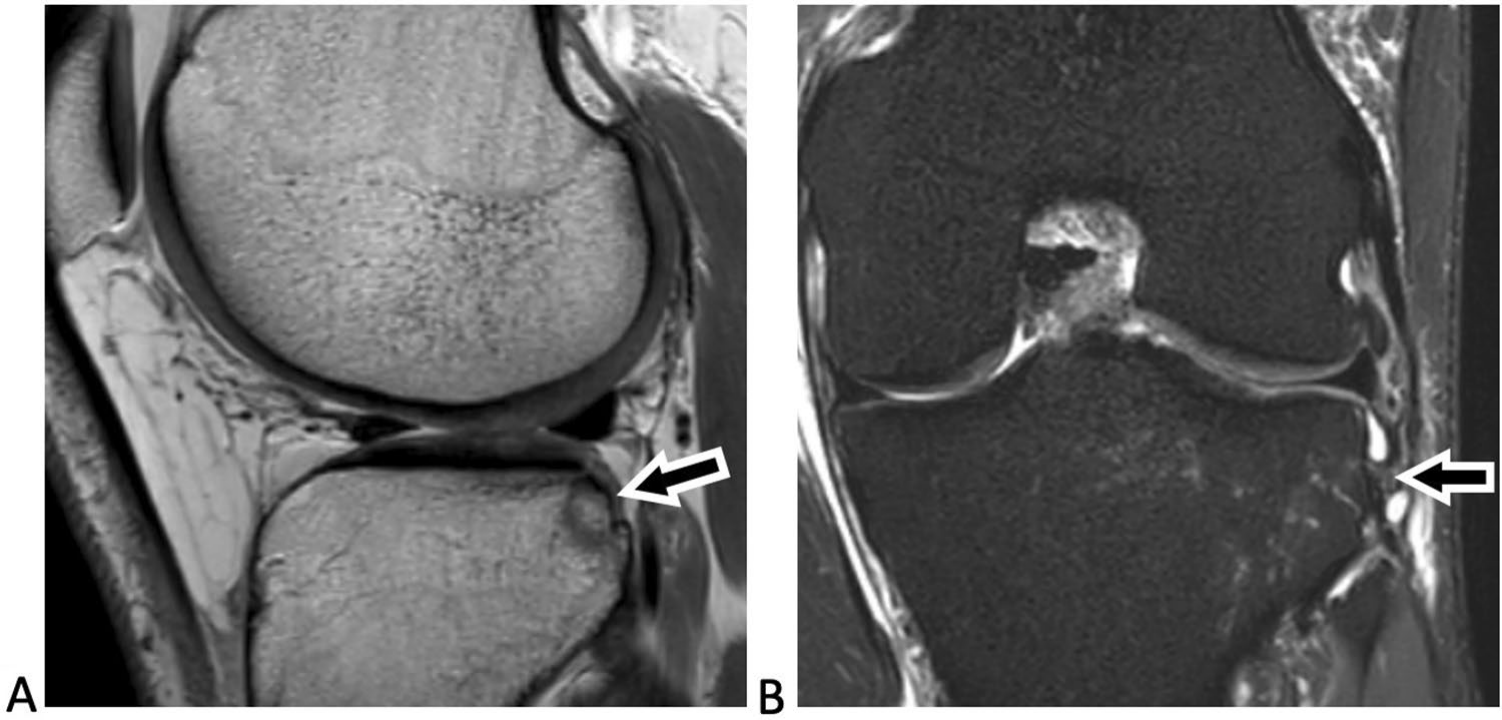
Magnetic resonance imaging of the left knee joint of a 43-year-old patient with an acute ACL injury. The sagittal proton-density-weighted image (A) shows a posterolateral tibial plateau fracture with an impacted fragment (arrow in A), corresponding to a Type IIIb fracture. The coronal T2-weighted fat-suppressed image (B) shows hyperintense signal alteration with partial tearing of the distal fibres of the anterolateral complex (arrow in B), consistent with a high-grade injury
